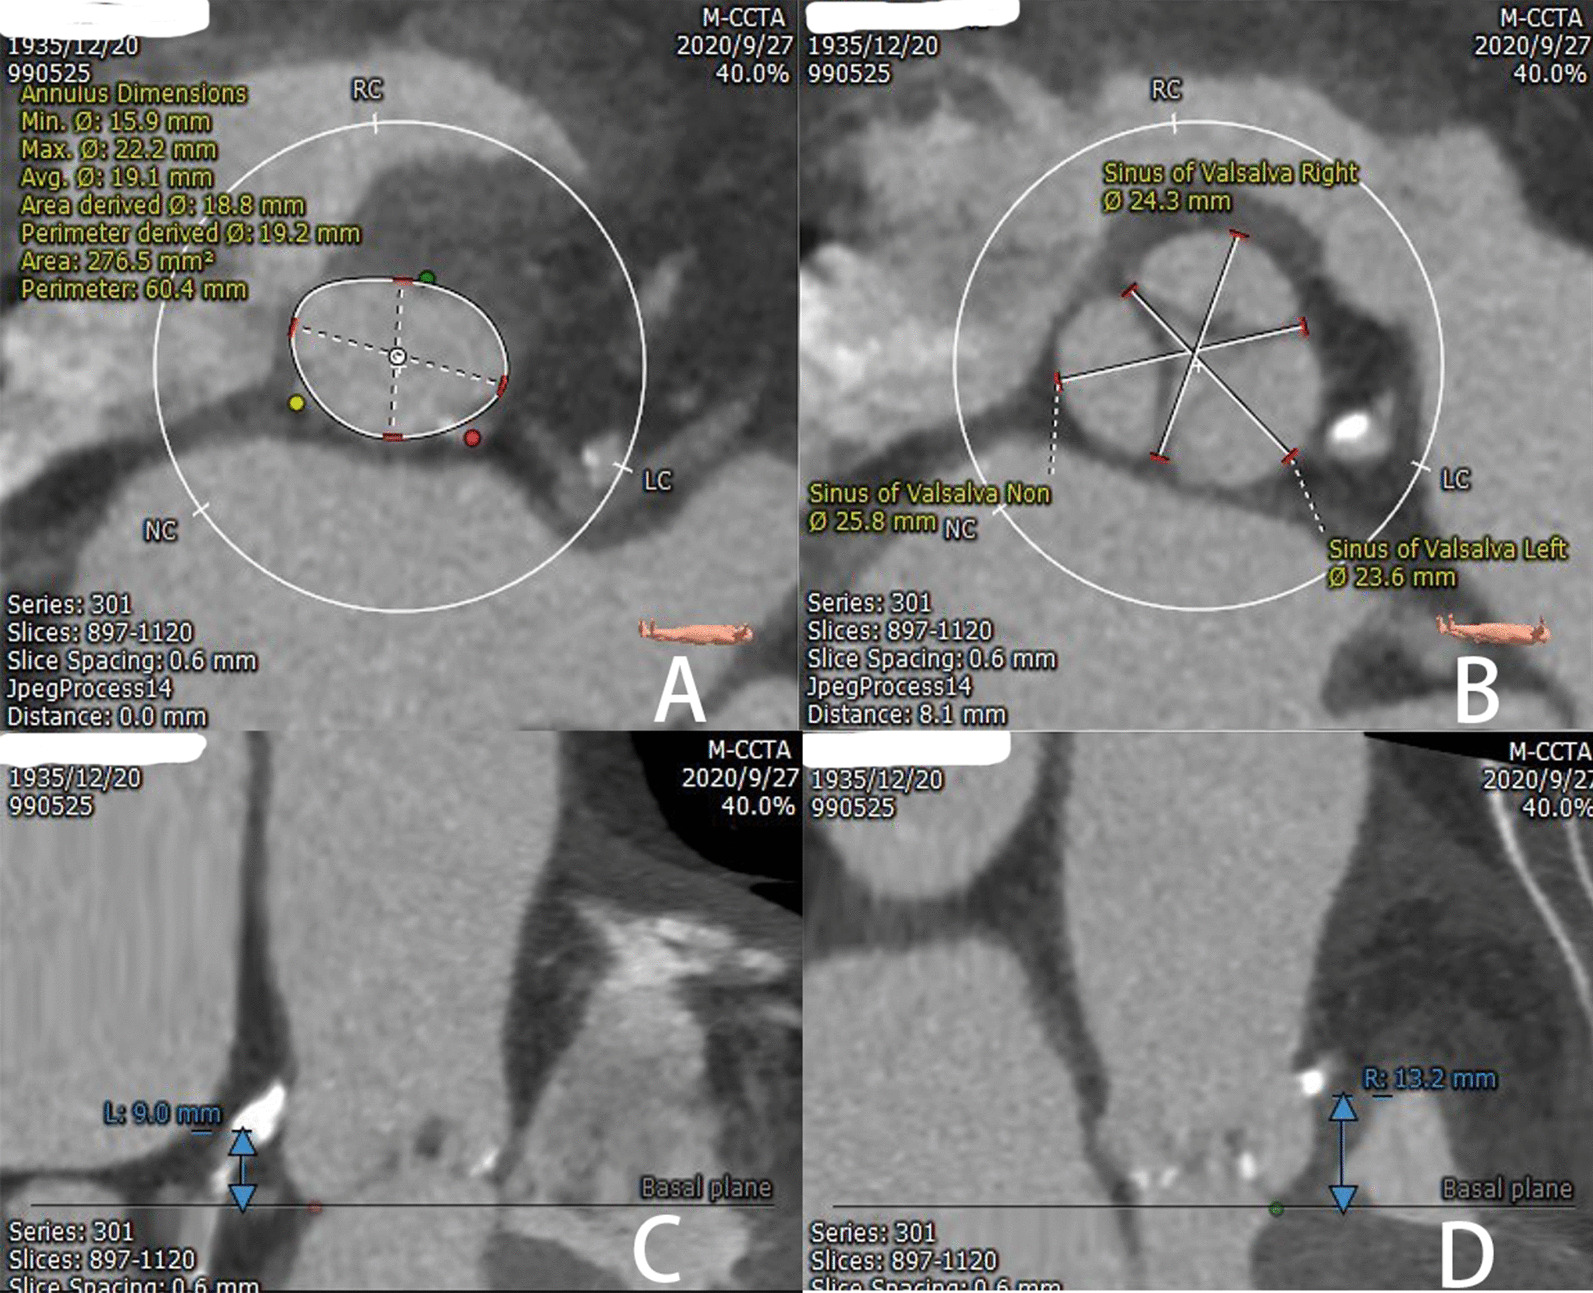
The measurements of CT scan. a Annulus diameters and perimeter; b Sinus of Valsalva depths; c Left coronary height; d Right coronary height
